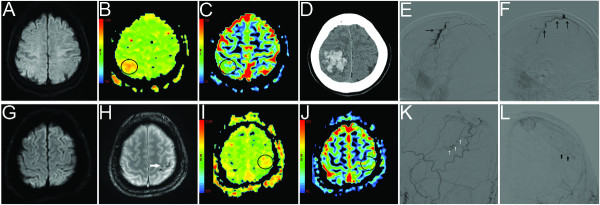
Brain MRI, brain CT, and digital subtraction angiography (DSA) of the two cases. Case 1. The baseline diffusion-weighted imaging (DWI) revealed no signal changes (A), while the perfusion-weighted imaging (PWI) showed a mean transit time (MTT) delay [interhemispheric ratio (IR) 1.07] and increase of relative cerebral blood volume (rCBV; IR 1.04) in the right postcentral gyrus (B, C). After neurological deterioration, a brain CT revealed a multiple lobar ICH in the right fronto-parietal lobe (D). A lateral view of the external carotid artery (ECA) on digital subtraction angiography (DSA) revealed a right middle meningeal artery-vein fistula with cortical venous reflux (E, F). Case 2. The baseline DWI showed no signal changes (G), whereas there were signal void signs in the left parietal cortex on the gradient echo MRI (H) and increase of MTT (IR 1.04) and rCBV (IR 1.24) on the PWI in the left paracentral sulcus area (I, J). Lateral (I) and frontal (J) views of the left ECA on DSA revealed arteriovenous fistula with single cortical venous reflux fed by the left middle meningeal artery.
